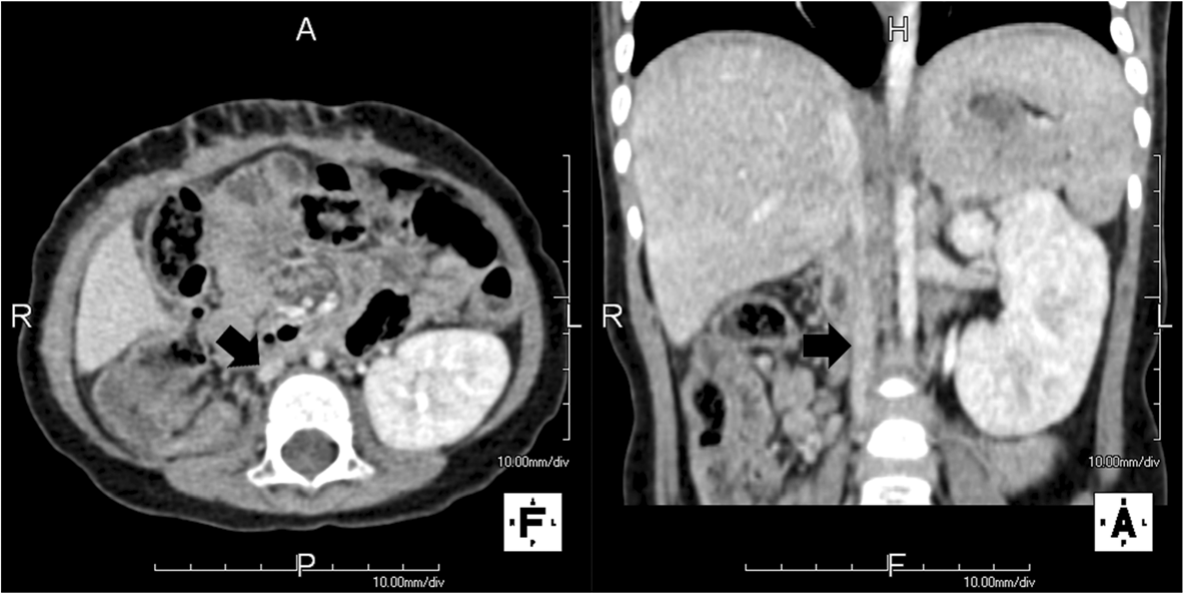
Axial and coronal computed tomography scan with contrast 3 months after operation showing the right inferior vena cava played a dominant role and the left inferior vena cava was not detected clearly. Straight arrow denotes the right inferior vena cava
